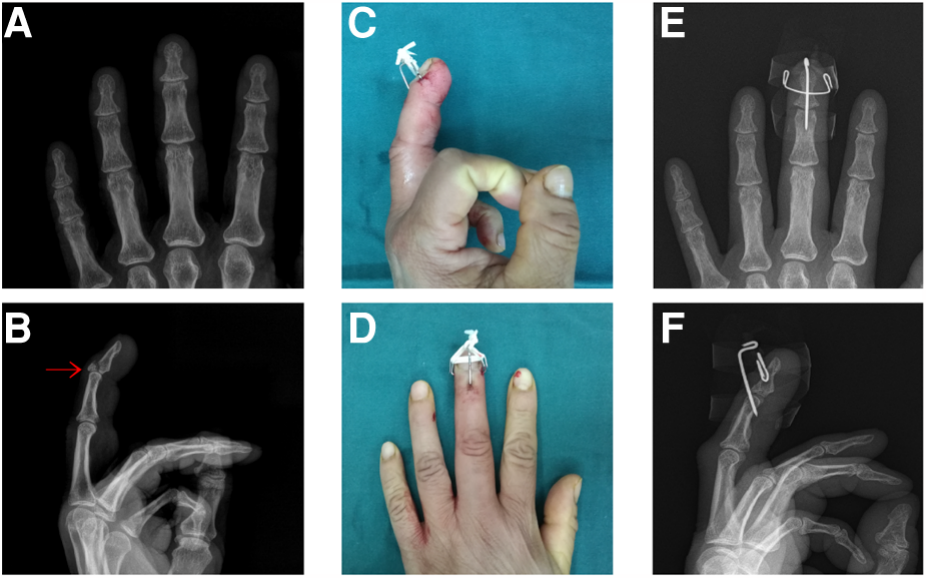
Preoperative and postoperative data of patient No.7. (A,B). Preoperative X-ray. (C,D). Postoperative X-ray. (E,F). Postoperative appearance.
